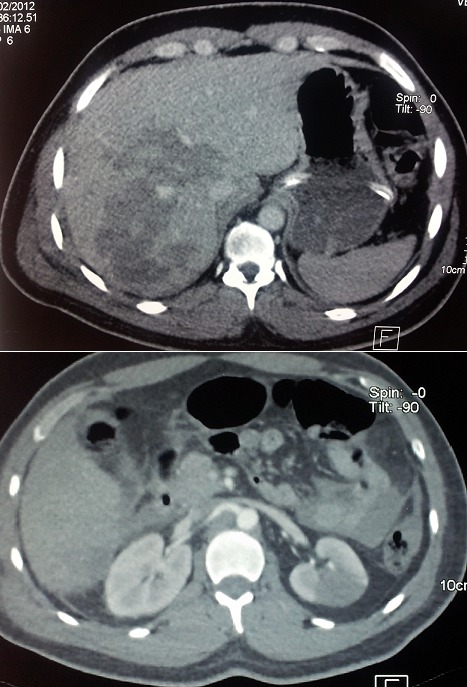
Abdominal CT scan revealed multiple liver and kidney contusions
